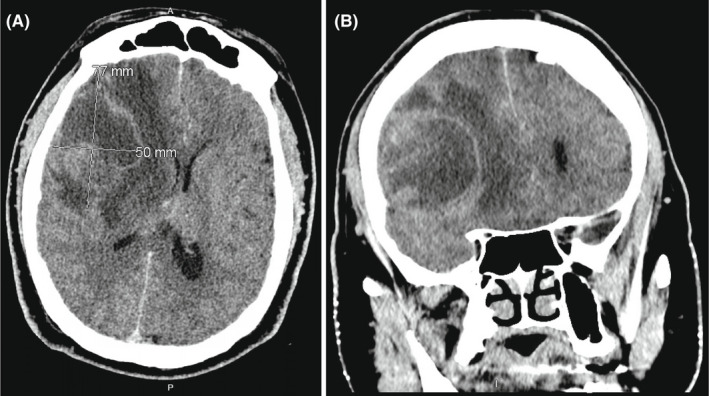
Axial (A) and coronal (B) head CT with contrast outlines a partially and peripherally enhancing right frontal lesion, measuring 77 × 50 × 61mm
